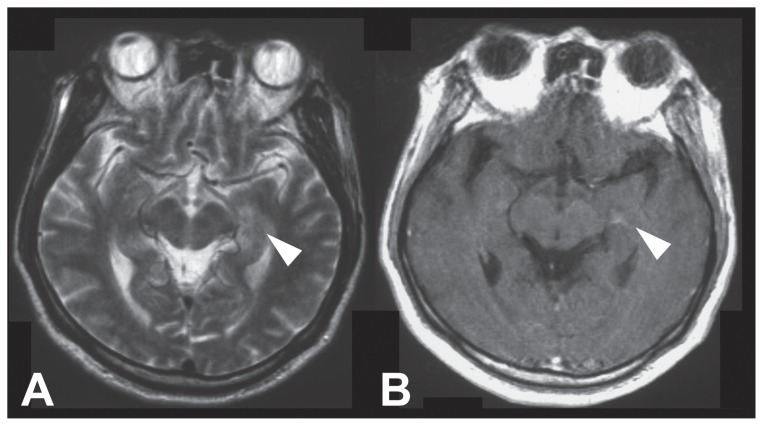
Brain magnetic resonance imaging. A) A T2-weighted image, showing increased signal intensity in the left medial temporal lobe. B) A contrast-enhanced T1 sequence, showing abnormal enhancement in the same area. After intensive care, these abnormal signals disappeared.
